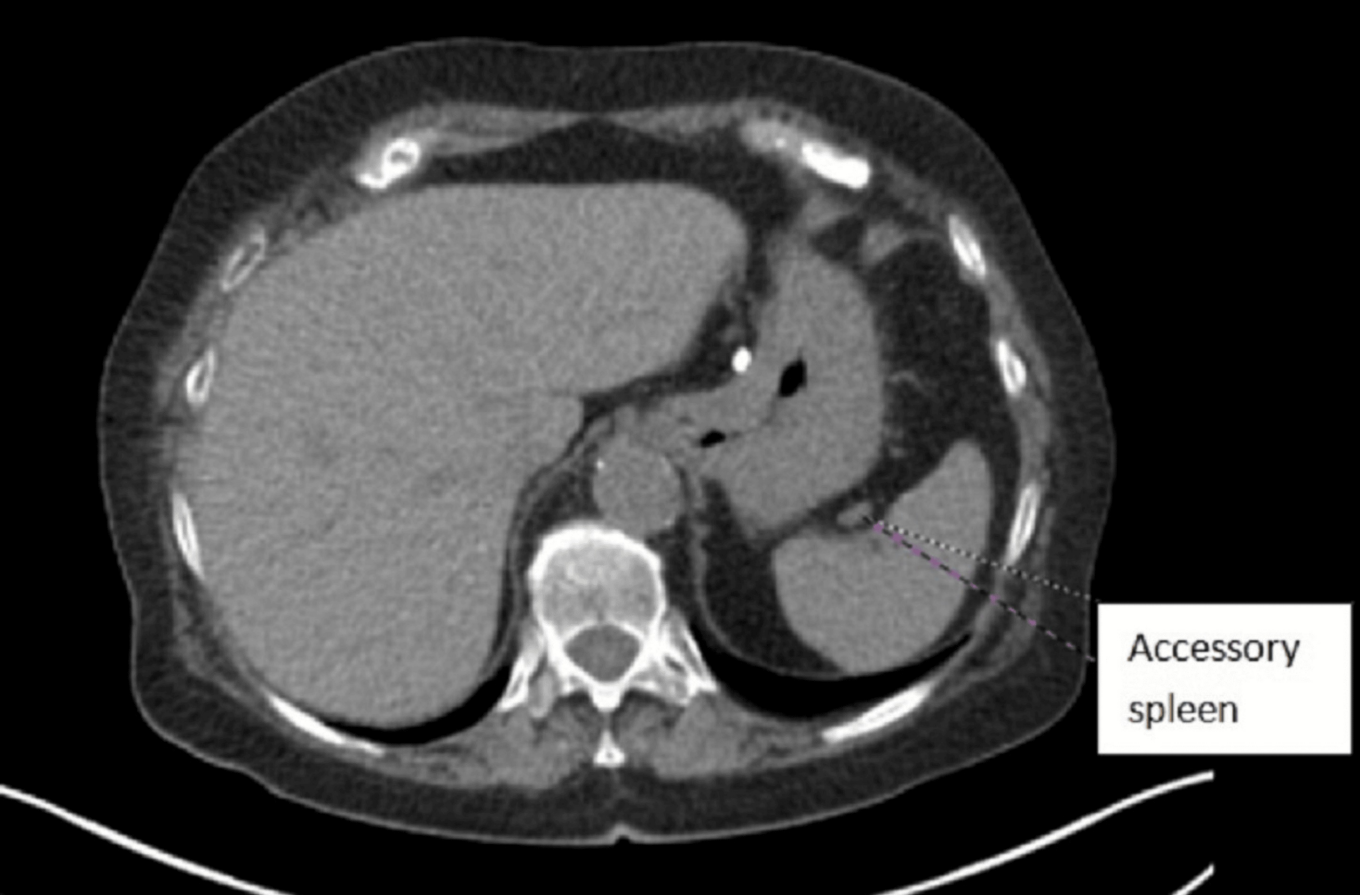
A CT scan of the flattened formation occupying the splenic hilum which was considered as accessory spleen
